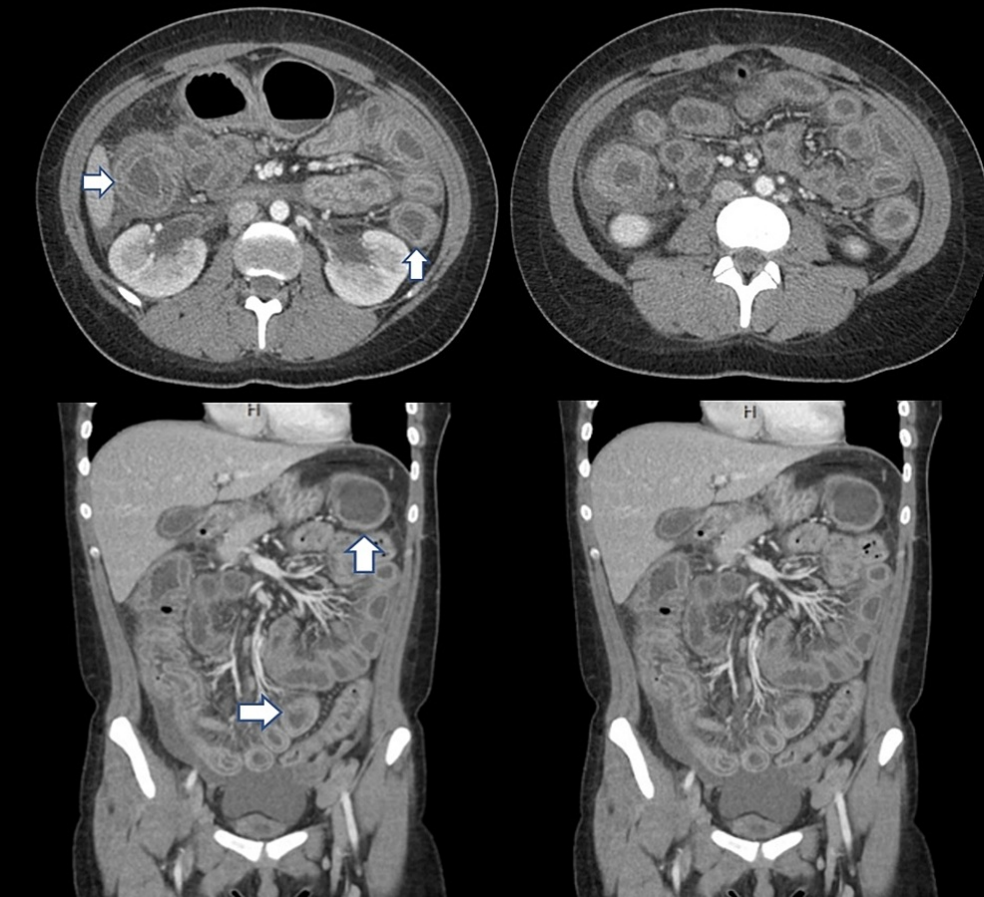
Contrast-enhanced abdominal CT scan - case 1 The scan revealed “target” or “double halo” sign (arrows) that represent circumferential thickening of the bowel wall secondary to submucosal edema. Both small and large intestines were affected in this case CT: computed tomography
